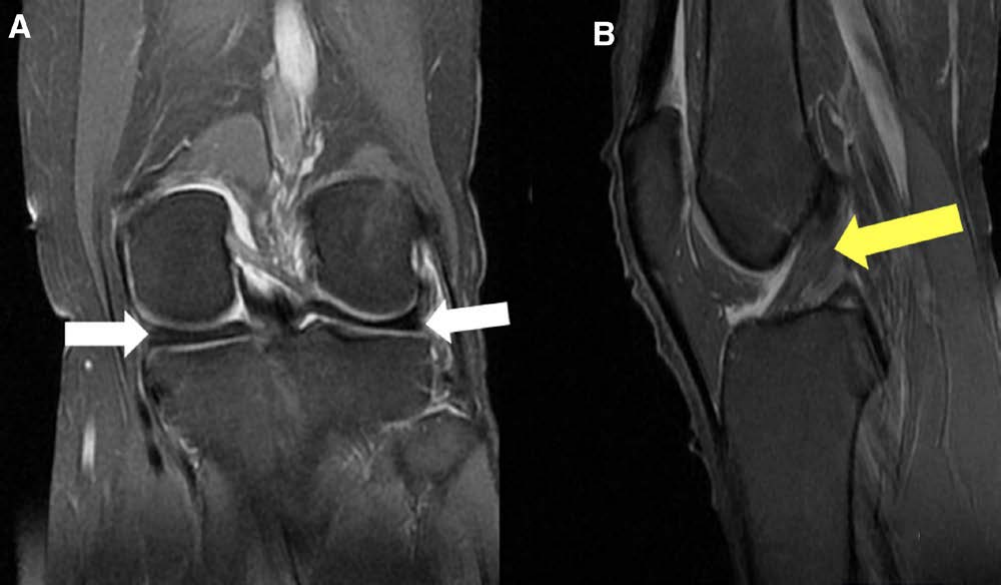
Coronal (A) and Sagittal (B) MRI cuts demonstrating intact menisci (white arrows) and ACL (yellow arrow). ACL = anterior cruciate ligament, MRI = magnetic resonance imaging.
